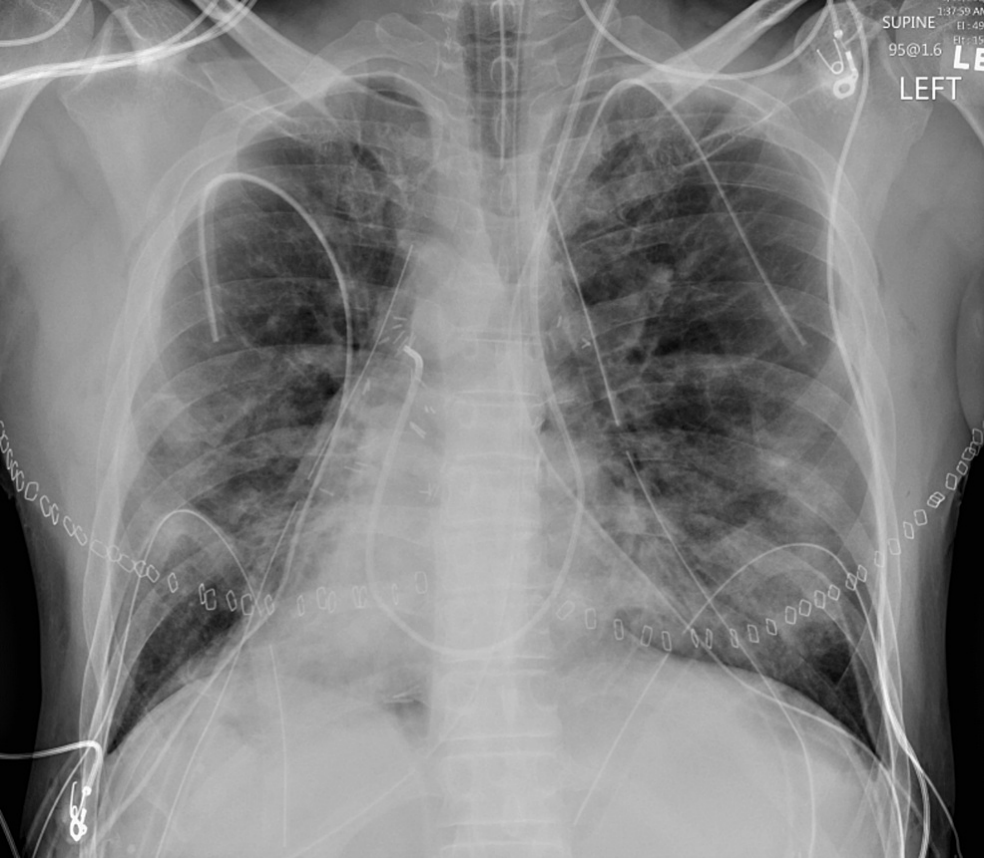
Chest x-ray post operatively shows newly implanted lungs.
